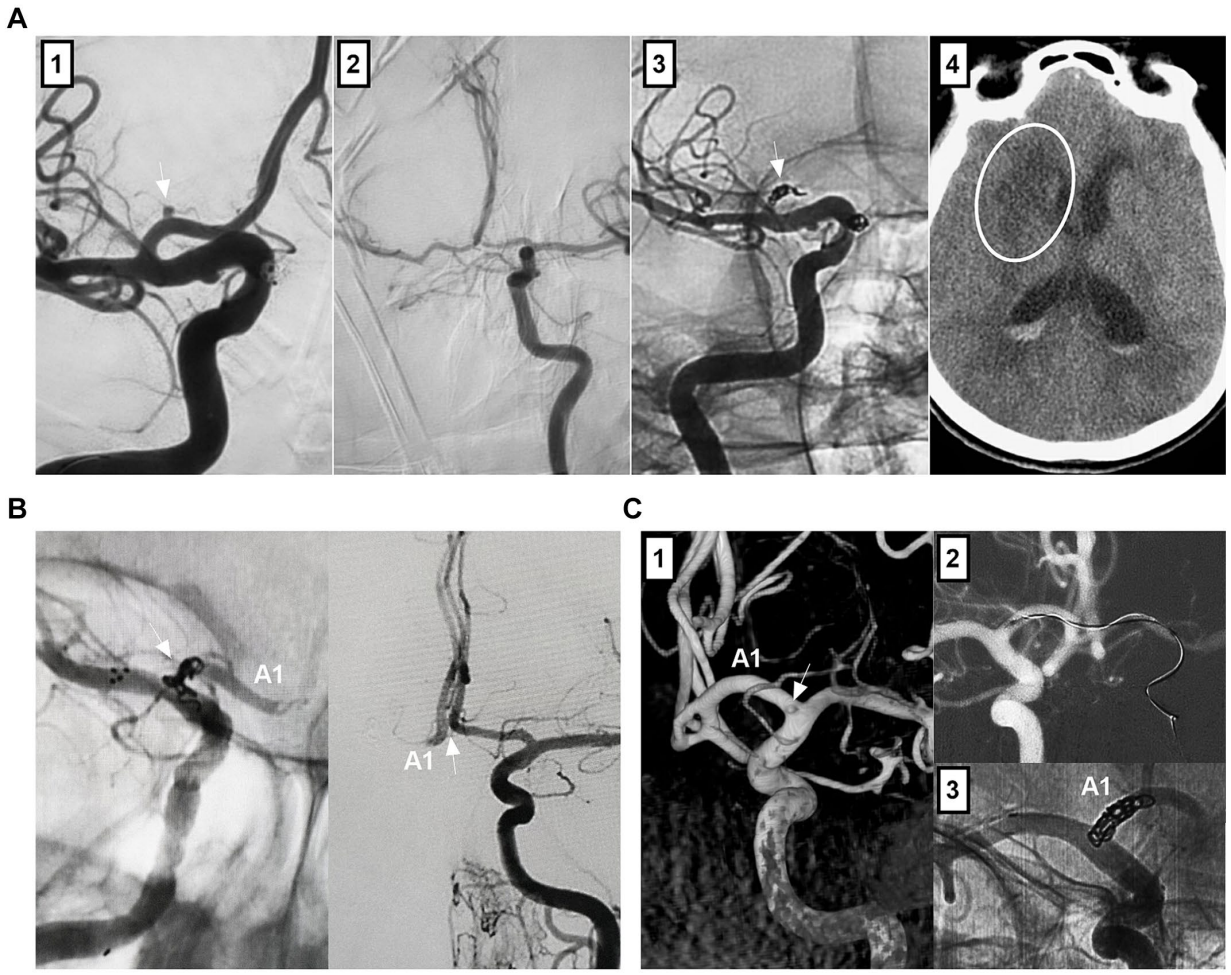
Treatment of A1 aneurysms by parent artery occlusion. (A) Panel 1: DSA showing an A1 aneurysm (arrow); Panel 2: DSA showing the compensation of the blood flow from contralateral ICA via the competent AcomA; Panel 3: Unsubtracted DSA showing the coiling (arrow) of A1 segment; Panel 4: Postoperative CT showing the infarction (circle) of head of caudate nucleus. (B) Left panel: Unsubtracted DSA showing that A1 aneurysm was coiled together with the A1 origin (arrow) by ipsilateral ICA approach; Right panel: DSA of contralateral internal carotid artery showing the patent AcomA (arrow). (C) Panel 1: 3D-DSA showing that a tiny A1 aneurysm; Panel 2: Roadmap image showing the contralateral approach to occlude the A1 aneurysm; Panel 3: Unsubtracted DSA showing that A1 aneurysm was coiled together with the A1 origin. 3D, three-dimensional; A1, first segment; ACA, anterior cerebral artery; AcomA, anterior communicating artery; CT, computed tomography; DSA, digital subtracted angiography; ICA, internal carotid artery.
